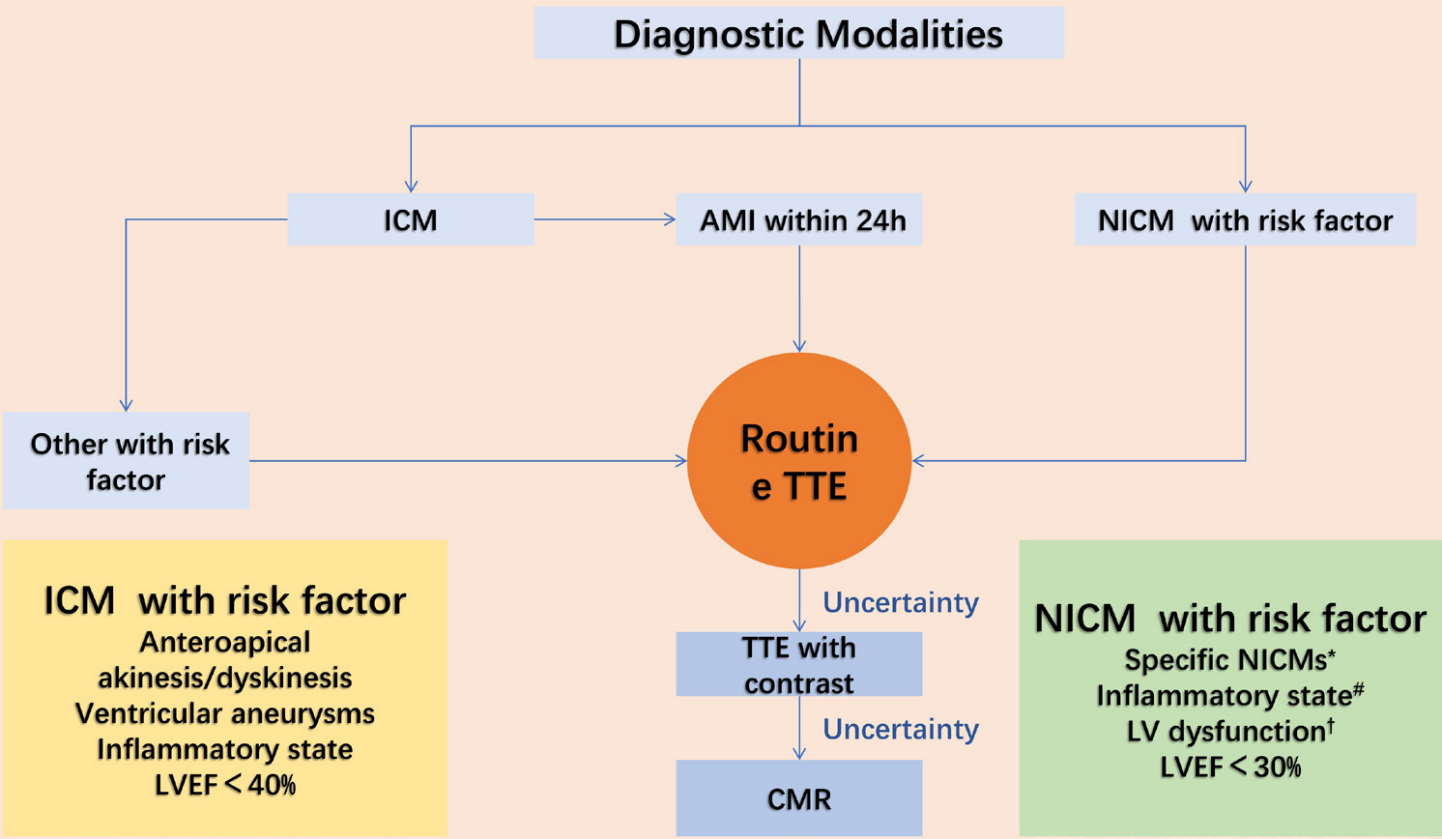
Proposed diagnostic modalities for left ventricular thrombosis. AMI, Acute myocardial infarction; CMR, cardiovascular MR; ICM, ischaemic cardiomyopathies; LV, left ventricular; LVEF, left ventricular ejection fraction; NICM, non-ischaemic cardiomyopathies; TTE, transthoracic echocardiography. *Dilated cardiomyopathy, Takotsubo syndrome, left ventricular myocardial densification insufficiency, eosinophilic myocarditis, perinatal cardiomyopathy, or cardiac amyloidosis. #C reactive protein, fibrinogen and the neutrophil-lymphocyte ratio. †Reduced ejection fraction and/or large apical or anterior LV akinesis or dyskinesis (ie, aneurysm) and reduced contractile function facilitating stasis caused by an abnormal vortex.
